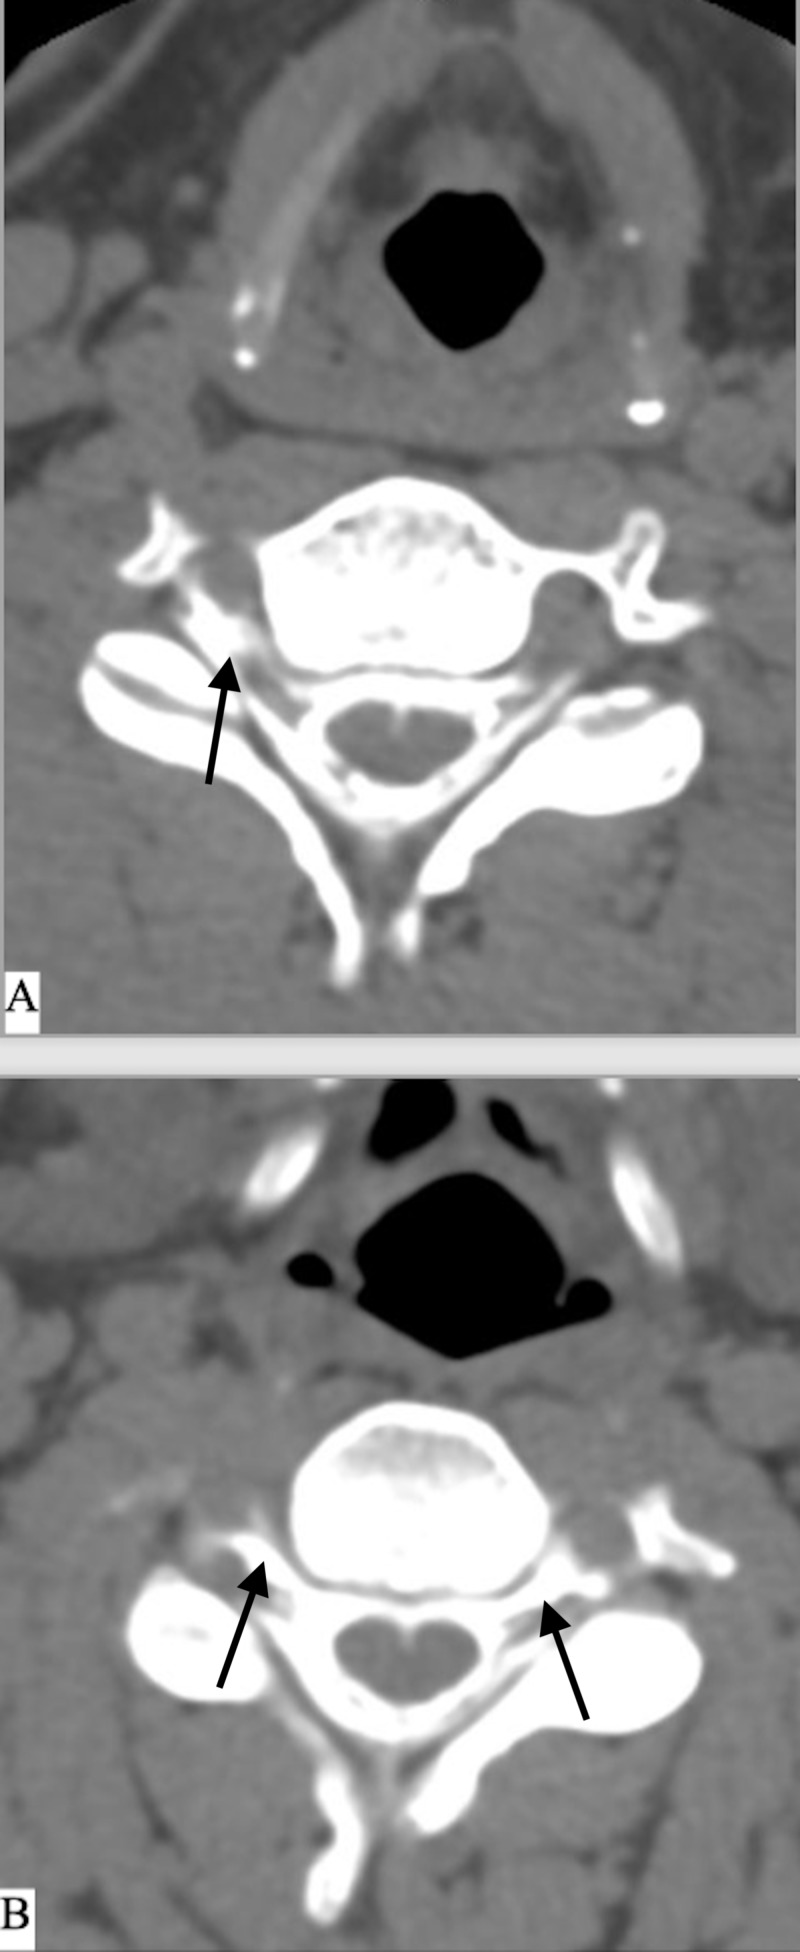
CT myelogram of the cervical spine A) Depicts the C4 level with contrast extravasation along the bilateral nerve roots. B) This is the C5-6 level with contrast extravasation predominantly along the right nerve root.
